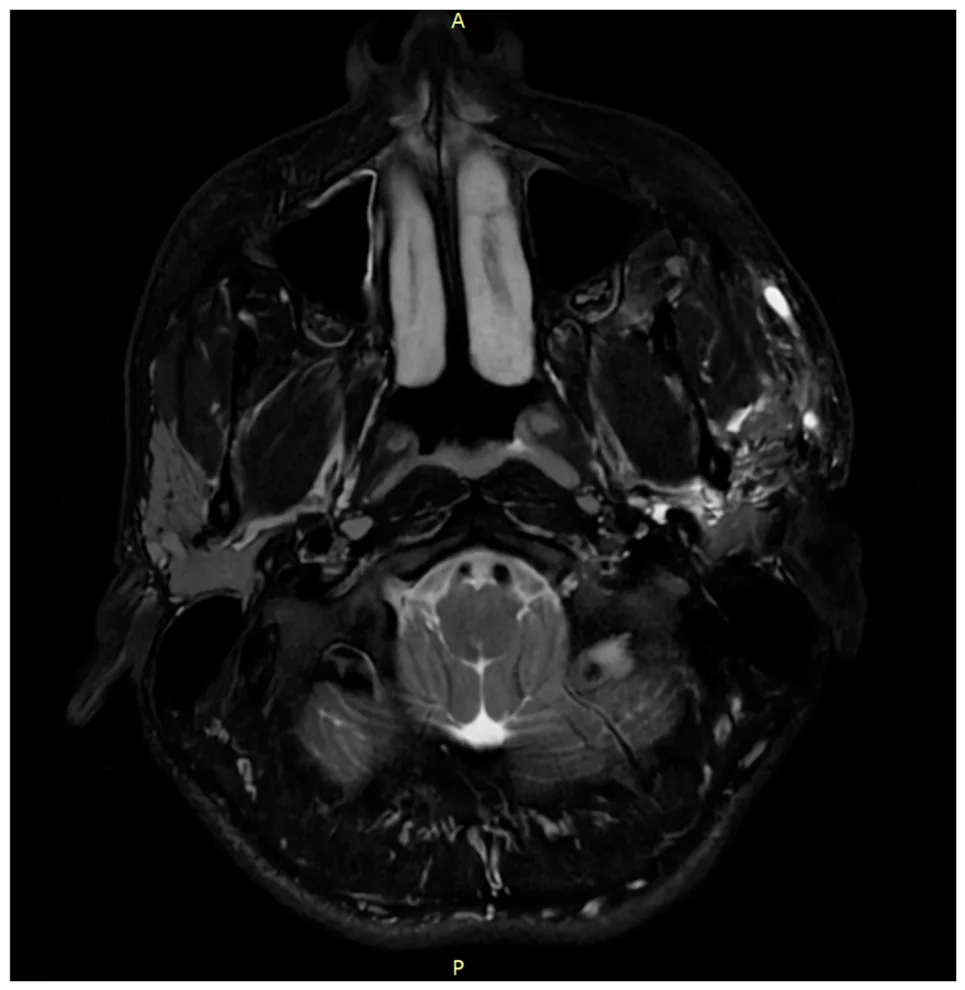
Axial T2-weighted fat-saturated magnetic resonance image 15 months after treatment. There are no features of local recurrence. Residual bands of lymphatic malformation tissue are visible in the region of the left parotid gland.
